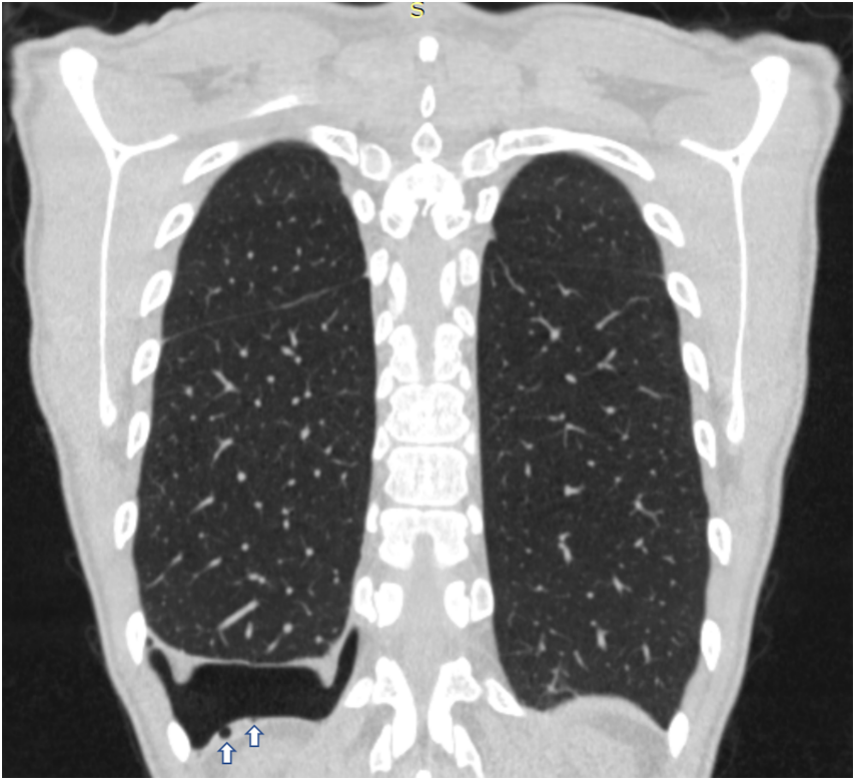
Coronal chest CT scan of a patient with CP showing on the right side small diaphragmatic defects, called “air-filled bubble” perforations (indicated by white arrows). CT, computed tomography; CP, catamenial pneumothorax.
